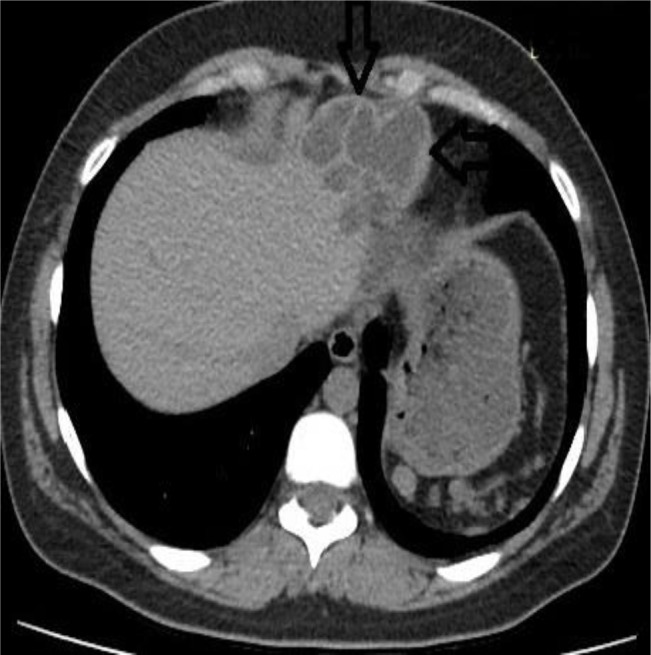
Axial CT image of the patient at first admission, shows a non-complicated type III hydatid cyst in left hepatic lobe (Arrow)
